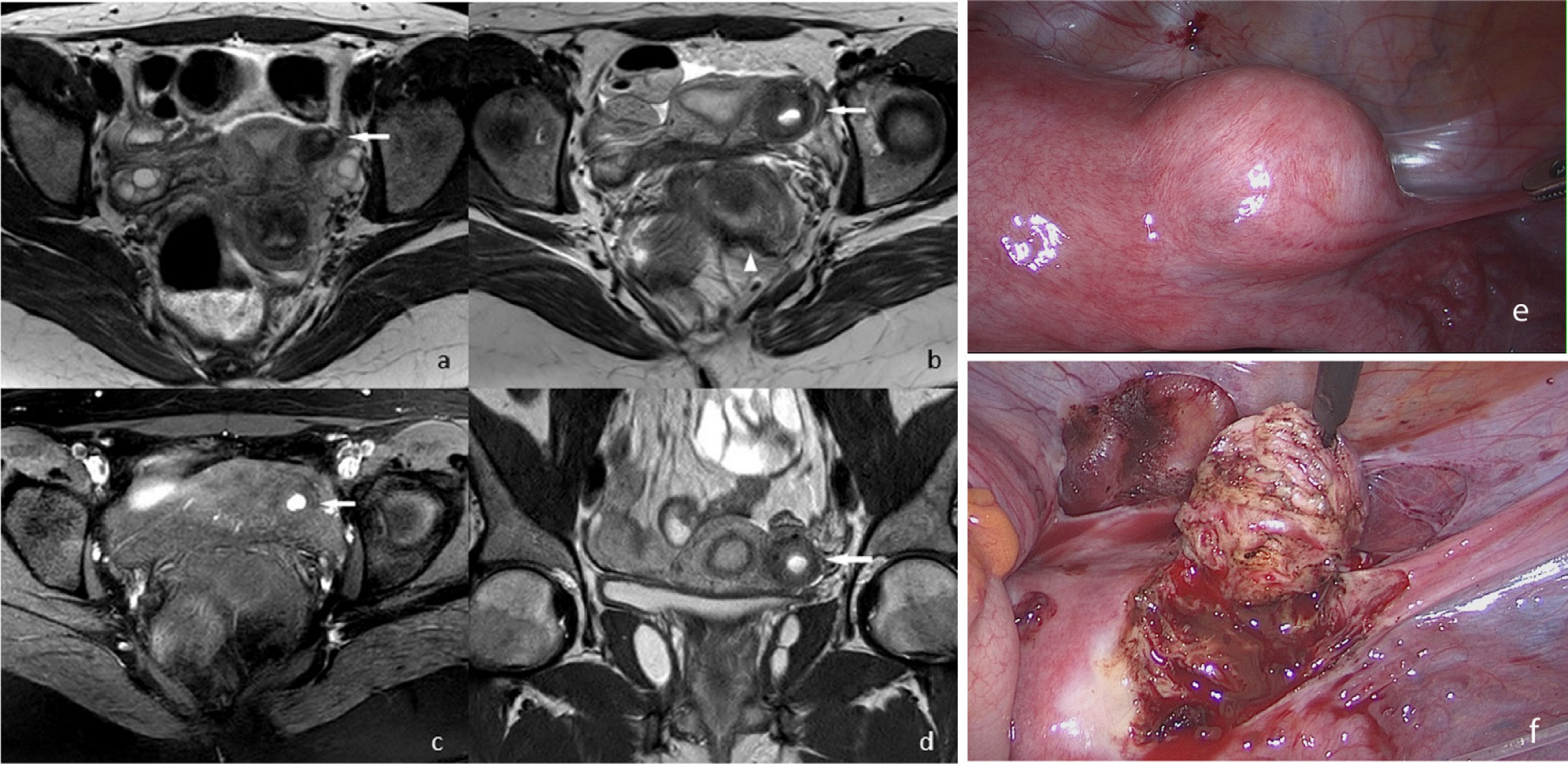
A 23-year-old nulliparous young woman referred for chronic major dysmenorrhea. a: T2-weighted seque ce - axial view. First examination performed in 2015. The lesion is small (15 mm) with a small inner cystic part surrounded by a hypointense annular tissue. b: T2-weighted sequence - axial view. Second examination performed in November 2020. The lesion had increased in size, now measuring 30mm. The hyperintense inner part presented a fluid-fluid lesion meaning different age for the blood. The lesion is located in the left part of the uterus near the origin of the round ligament (↑white arrow). The heterogenous inner part indicated the presence of blood clots. It was also found a thickening of the inner part of the left uterosacral ligament (▲white arrow head) c: T1-weighted sequence – axial view. The hypersignal inside the inner part of the lesion is larger than previously (↑white arrow). d: T2-weighted sequence -coronal view. It demonstrates the precise localization of the lesion at the level of the left uterine horn (↑white arrow). e: View of the laparoscopic surgery performed in February 2021. Intraoperative appearance of the mass located on the left side of the uterus and extending toward the left side of the broad ligament with smooth border.f: View of the laparoscopic surgery performed in February 2021. After incision, the chocolate-colored fluid was drained and the lesion was excised from surrounding myometrium. (Color version of the figure is available online.)
